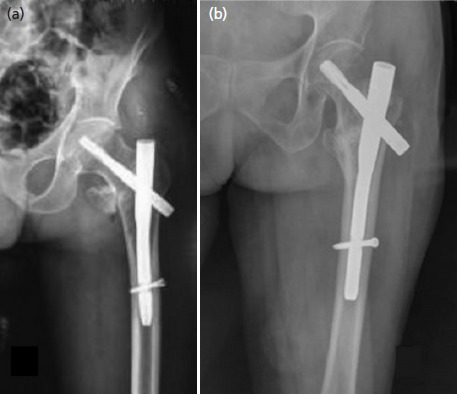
(a, b) Antero-posterior radiographs of two different patients showing union at the fracture site with protrusion of helical blade beyond the lateral cortex. At follow-up both patients still complain of thigh pain with tenderness over the blade insertion site.
